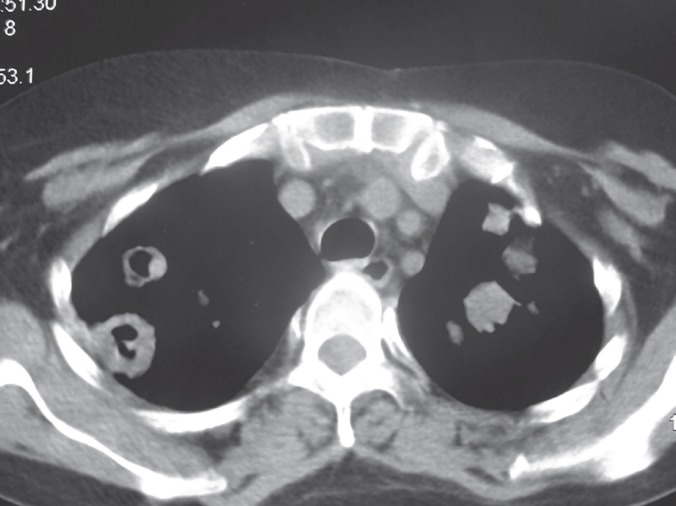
Computed tomography showed multiple consolidation with halo sign of both lungs
